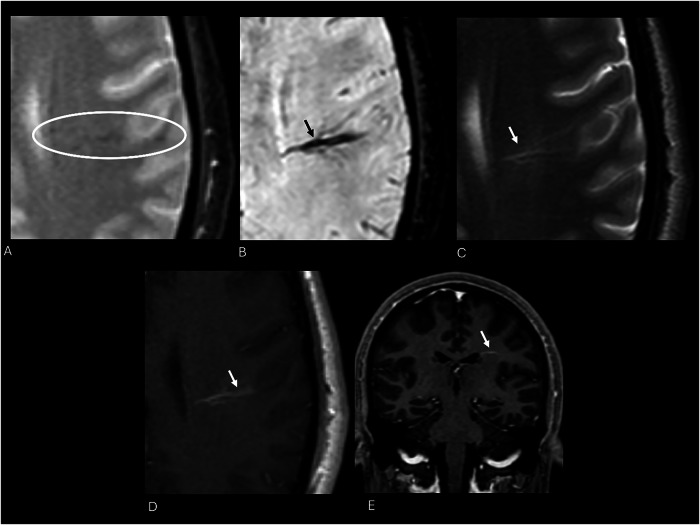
MRI performed for a 40-year-old male patient with status epilepticus. T2* image displays a significantly noticeable hypointensity in the left frontal lobe (white circle) (A), which becomes evident on SWI as a linear flow-void signal (black arrow) (B). On axial T2 turbo spin‑echo (TSE), the anomaly shows a branched vascular structure with high signal intensity, with subcortical involvement (white arrow) (C). Post gadolinium axial 2D T1-W spin‑echo (SE) (D) and coronal 3D T1-W GRE (E) display the characteristic linear enhancement of the ectatic collector vein draining into subependymal veins of a DVA
